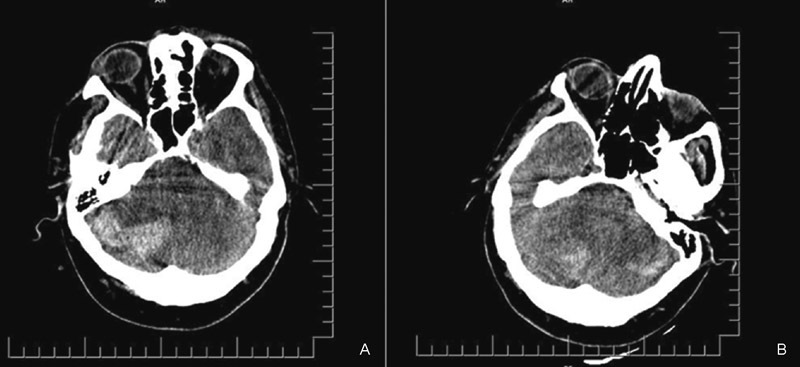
Postoperative cranial noncontrast computed tomography axial section. (A) Hyperdense lesion showing right cerebellar hemorrhage with moderate mass effect. (B) Bilateral cerebellar hemorrhage.
